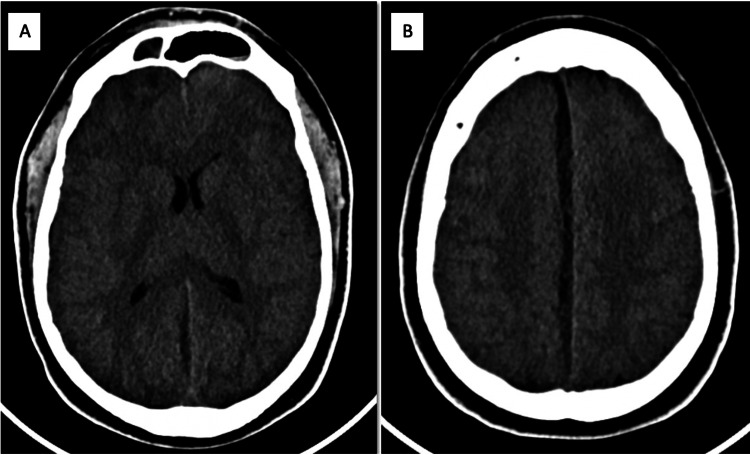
Initial CT without contrast showing right frontal hypodense fluid collection (A) and hypodense subdural collection in falx consistent with hematoma vs empyema (B)
